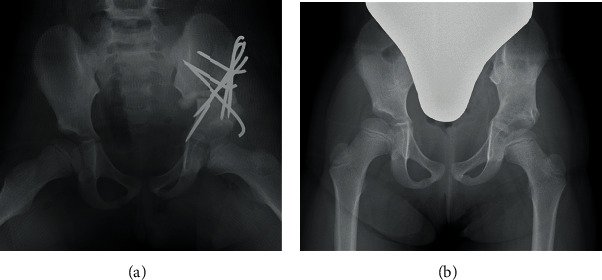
(a) The radiograph just after the additional surgery. (b) The radiograph of the hips at the last follow-up.
